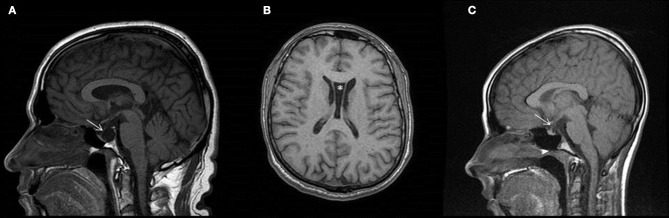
Brain MR images of the examined patients. T1 weighted images of Patient II/2 (A,B), and of Patient III/1 (C). Image (A) and (C) demonstrate empty sella on sagittal images. Infundibulum sign is identifiable (arrows), thus proving empty sella vs. cystic mass of pituitary.
